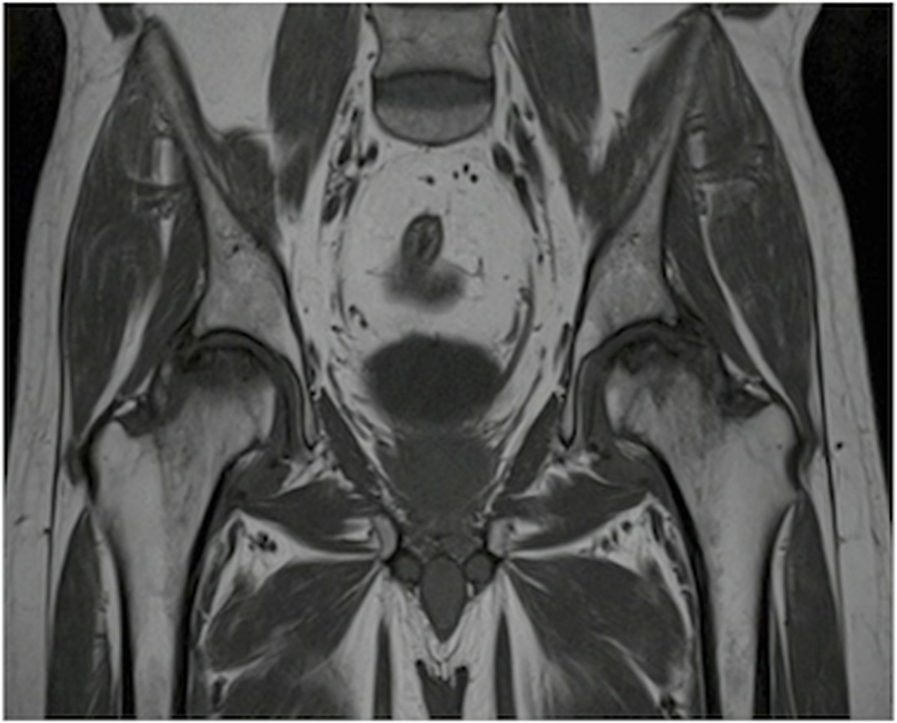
Preoperative MRI scan. T1-weighted coronal MRI scan demonstrating bilateral ON of the femoral head.
